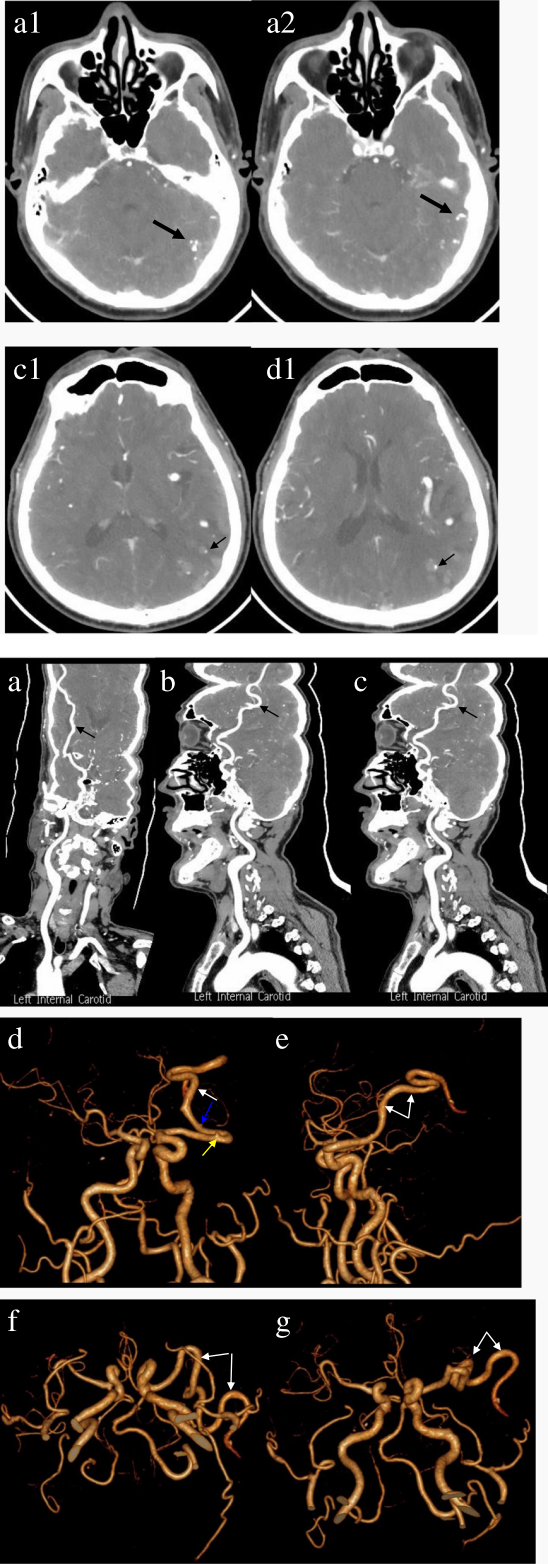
CT angiography (CTA) axial slices (a1, a2, c1 and d1) also showed calcification at the same positions of the head CT scan: a1 and a2 to a (thick black arrow), c1 to c and d1 to d (thin black arrow), and the vessels are not enhanced. CTA with curved planar reformation (a ~ c) and with 3D reconstruction (d ~ g) showing that the left MCA is huge and tortuous and almost the same diameter from the beginning to the end (black arrow in a ~ c and white arrow in d ~ g). One small branch (yellow arrow) from the M1 segment and another small one (blue arrow) from the M2 segment
